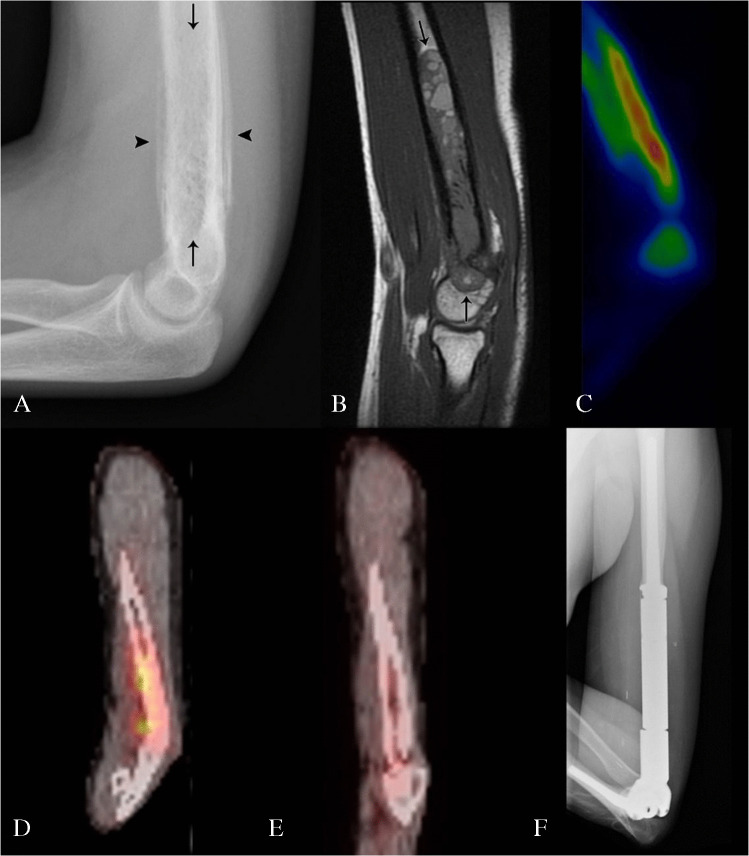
17-year-old male with Ewing sarcoma of the distal humerus. Lateral radiograph (A) demonstrates a permeative lytic lesion (arrows) with periosteal reaction (arrowheads). Sagittal T1 MRI (B) better shows the full extent of the lesion (arrows). Sagittal reformation of SPECT-CT Tc99m-MDP bone scan (C) displays intense tracer uptake within the lesion. Pre-treatment sagittal reformation of 18F-FDG PET-CT (D) reveals intense metabolic activity with an SUVmax of 4.8. After completing 9 weeks of chemotherapy with vincristine, doxorubicin and cyclophosphamide, sagittal reformation of 18F-FDG PET-CT (E) demonstrates resolution of the previously seen metabolic activity, indicating excellent treatment response. The patient subsequently underwent limb salvage surgery with wide resection, with a lateral radiograph (F) showing modular endoprosthesis placement
